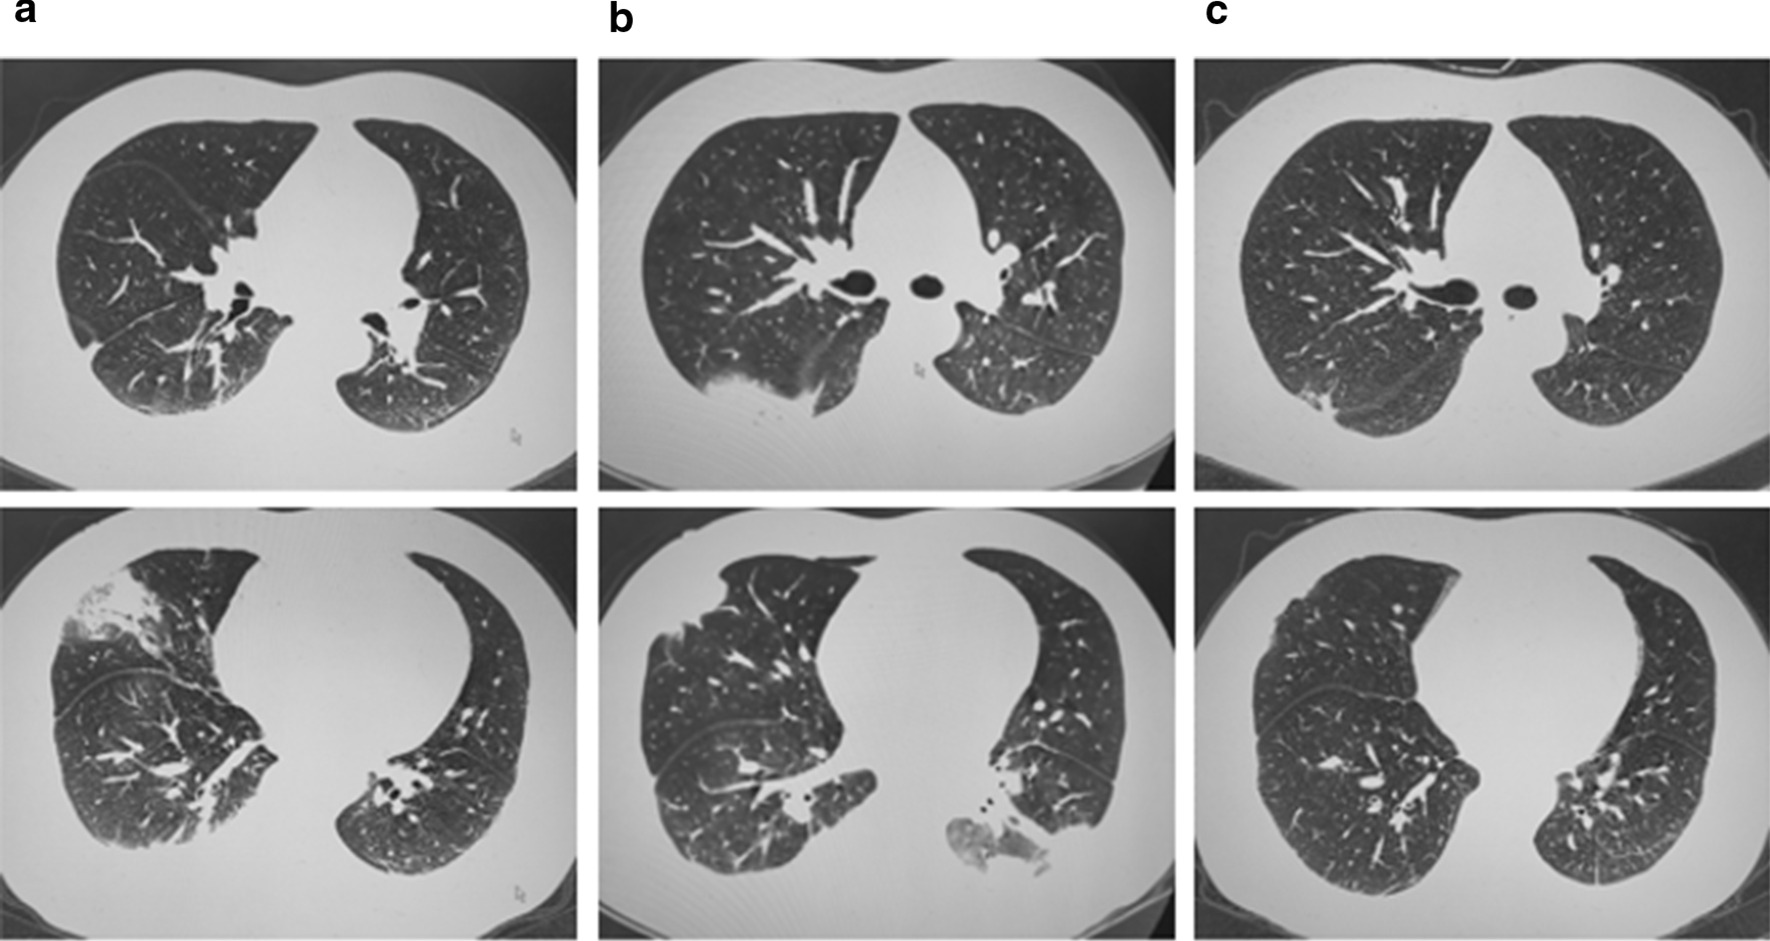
Chest CT scan (a). Upon arrival: chest CT imaging suggested pulmonary infection with pleural effusion (b). Seven days after admission: chest CT imaging suggested more extensive pulmonary infection with pleural effusion (c). Seventy days after admission: signs of lung infection were dramatically improved, and pleural effusion was also obviously absorbed
